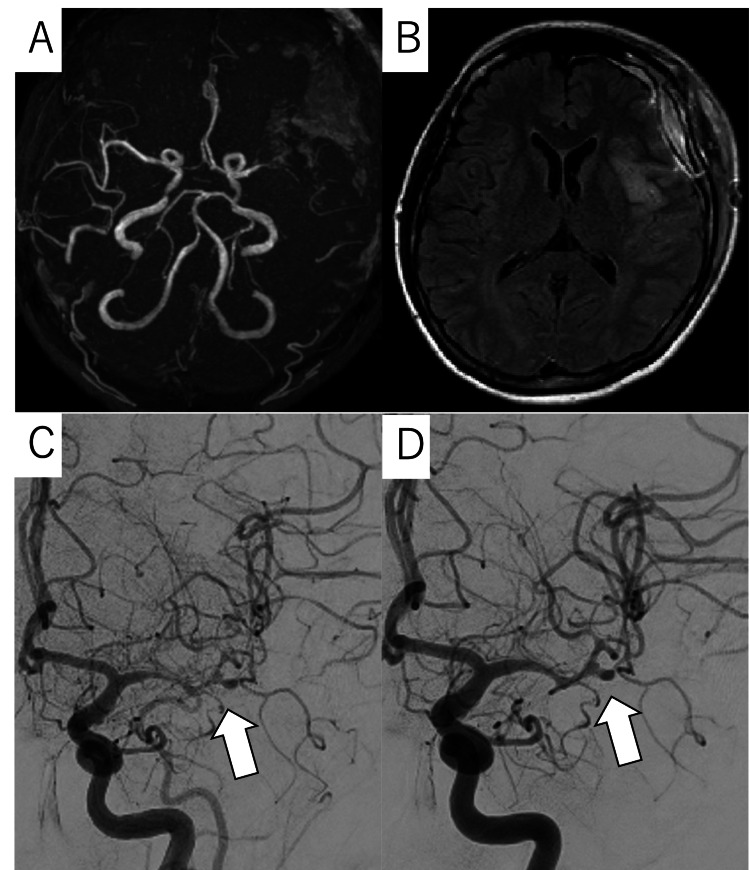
MRA, FLAIR, and DSA images on postoperative day 9 (A) Poor visualization of the left MCA; (B) Clarification and enlargement of ischemic lesions; (C) DSA before treatment revealed vasospasm in the left MCA territory (arrow); (D) DSA following intra-arterial fasudil injection showed improvement in the vasospasm (arrow) MRA, magnetic resonance angiography; FLAIR, fluid-attenuated inversion recovery; DSA, digital subtraction angiography; MCA, middle cerebral artery
